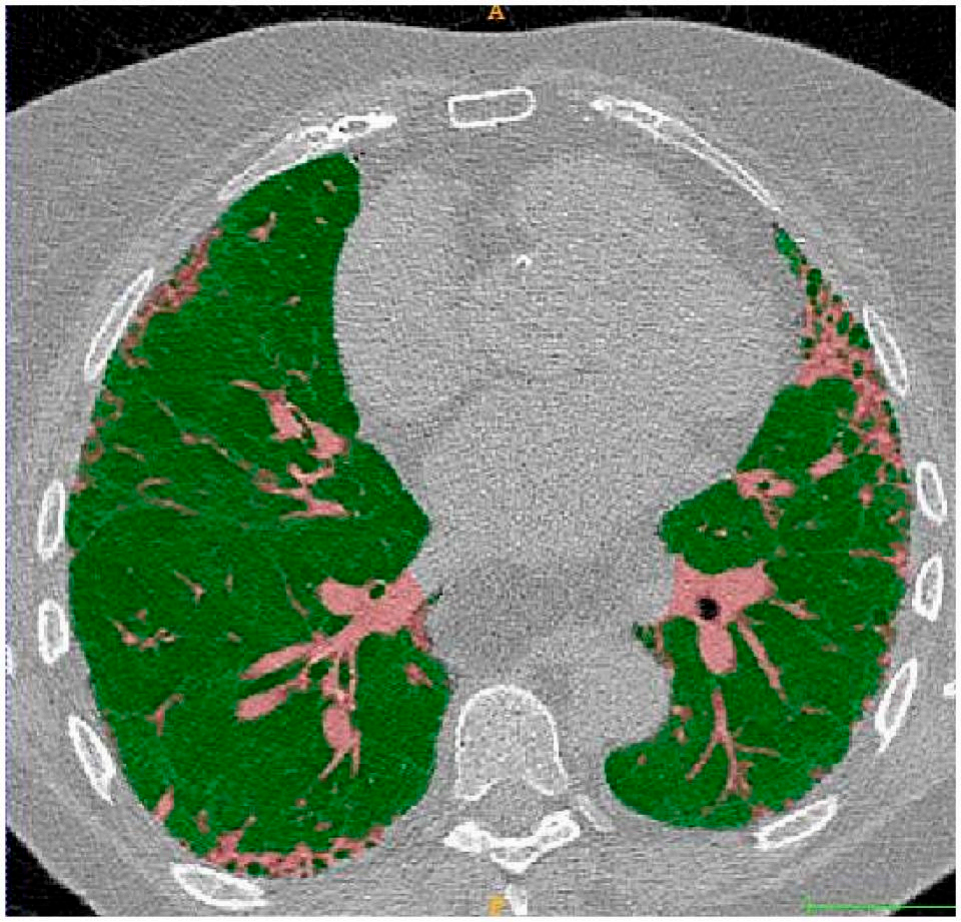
Brainomix. Axial HRCT in the lung parenchyma window, displaying the “weighted vascular-reticular score” (see text for details). Normal lung tissue is depicted in green, while vascular and reticular structures are segmented and quantified (pink). Courtesy of Dr. Peter George.
